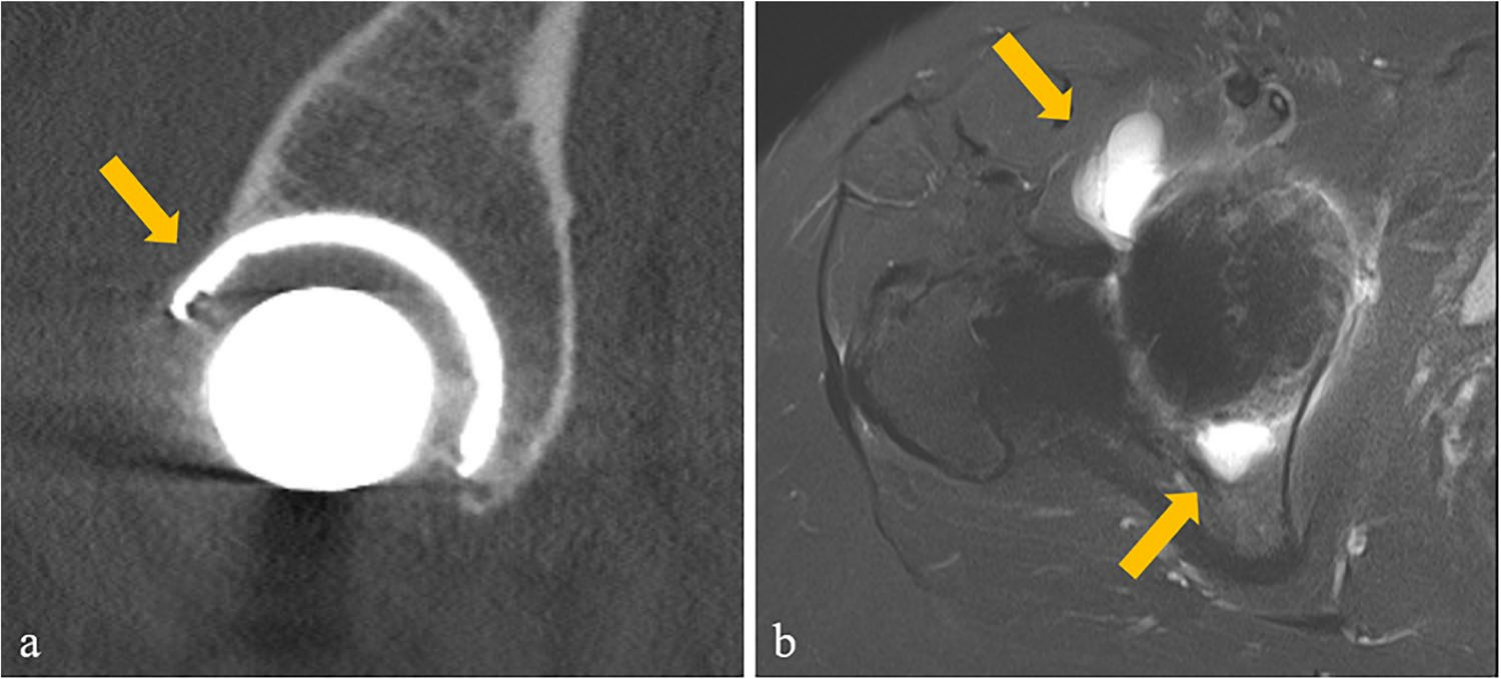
Exemplary images of a 64-year-old female patient with cup overhang and clinically diagnosed iliopsoas impingement following total hip arthroplasty. Sagittal CT images (a) clearly depicting the cup overhang. Axial STIR images of the right hip depicting fluid in the bursa iliopectinea as a sign of mechanical irritation of the iliopsoas tendon by cup overhang (b). STIR, short-tau inversion recovery
